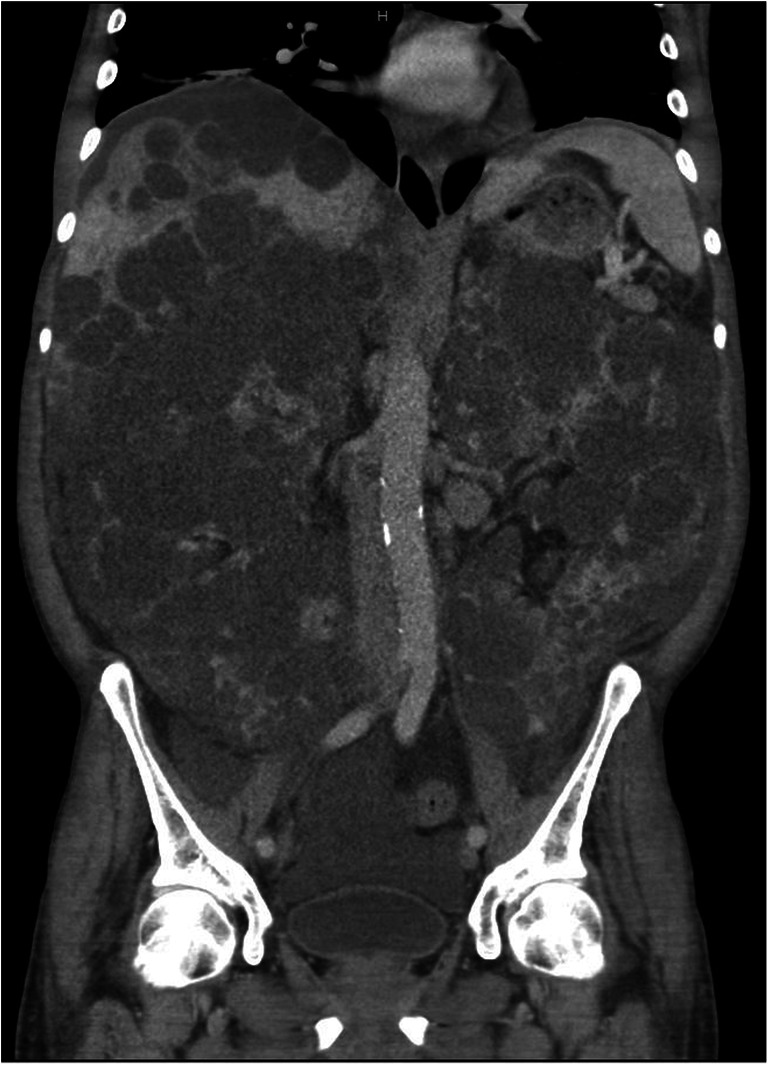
Coronal presentation of the caval region showing polycystic alterations in both kidneys on the CT scan (CT Revolution 256 slice, GE Healthcare, USA) before transplantation
